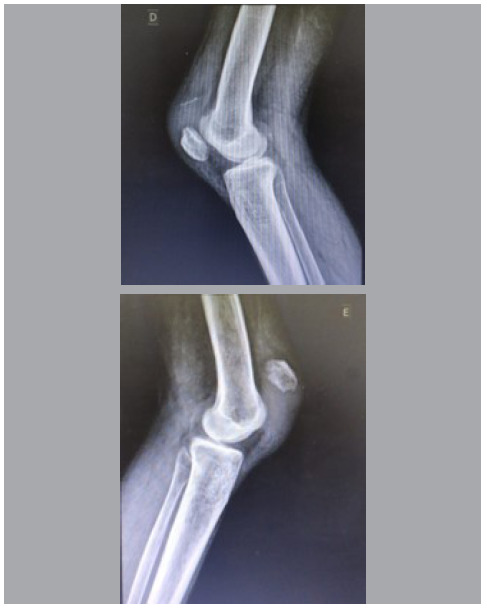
Radiographs showing patella baja on the right knee, with a Caton-Deschamps index of 2.1, and patella alta on the left knee, with a Caton-Deschamps index of 0.5.
